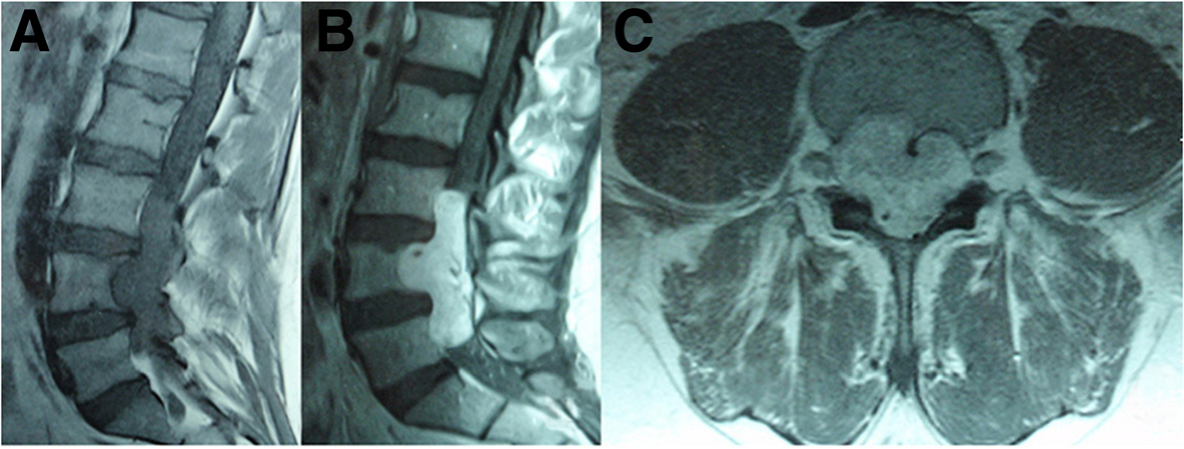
Pre-operative MR images of the lumbar spine revealed an intradural, extra-medullary, isointense mass behind the vertebral bodies of L3 to L5. The sagittal T1-weighted image showed the vertebral body of L4 exhibited a compressed deformation (A). The T1-weighted image showed homogenous enhancement with contrast (B). The axial T1-weighted image showed the vertebral body of L4 exhibited a compressed deformation (C).
